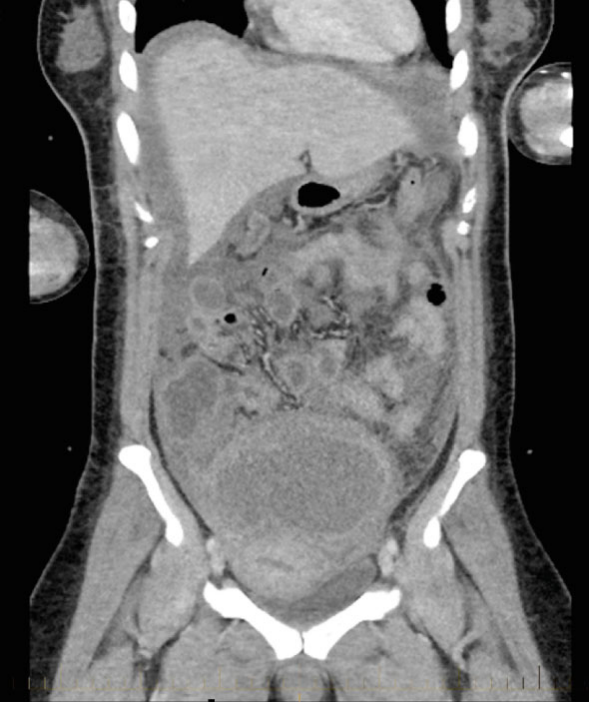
CT report of the abdomen and pelvis. There is a large cystic mass arising off the pelvis, which is complex in appearance. The wall is thick and irregular; this lesion has axial/transverse dimensions of 12.6 × 13.6 cm, with a sag/oblique long axis of 15.4 cm.
